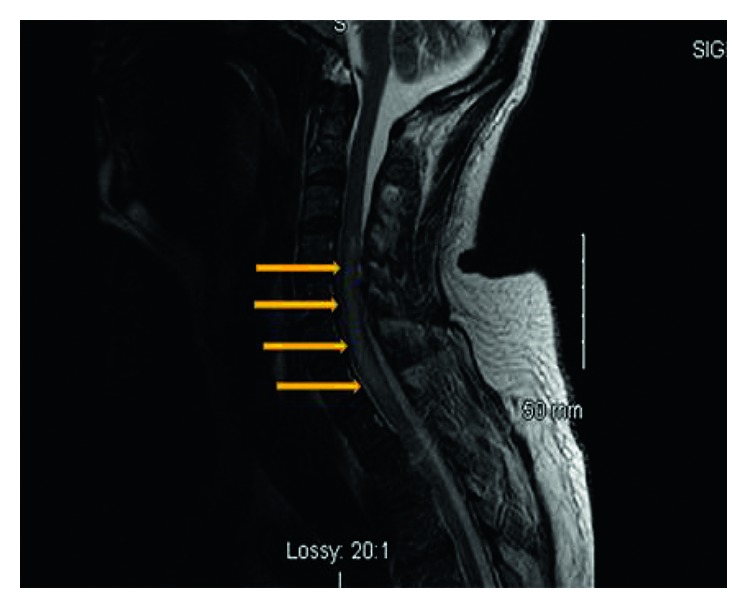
A sagittal T-weighted MRI of the C-spine of the patient on April 28, 2017, before the antifungal treatment. The arrows point to the spinal cord lesion due to coccidioidal infection.
